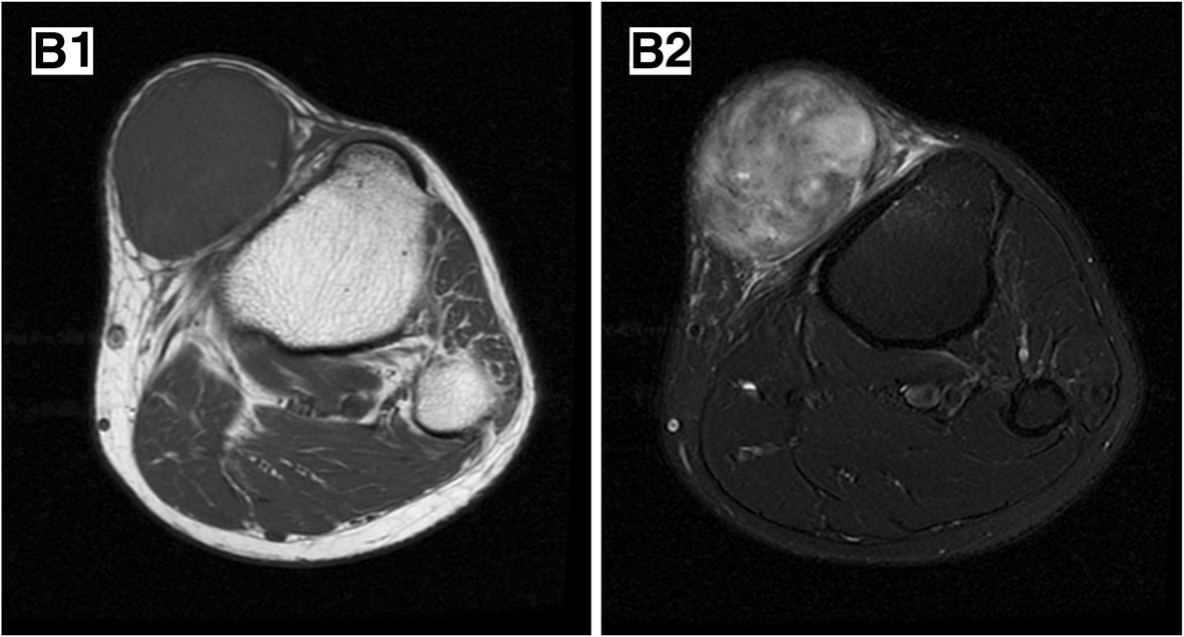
MPNST with NF-1 in a 49-year-old male. A well-defined, ovoid mass lies in the subcutaneous tissue, which demonstrates hypo-intensity on axial T1-weighted (B1) and obvious heterogeneous hyper-intensity on T2-weighted (B2) images. Perilesional edema and intratumoral lobulation are noticeable on T2WI
